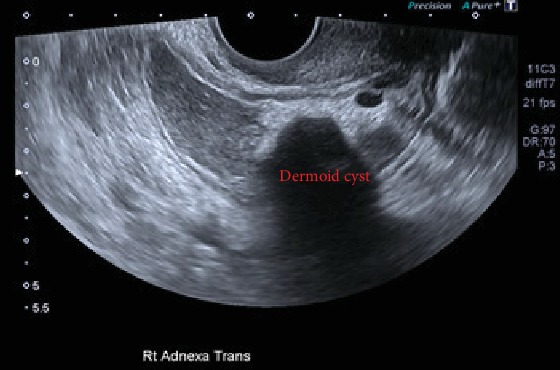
Ultrasound showing a large 10 cm well-circumscribed dermoid cyst.
